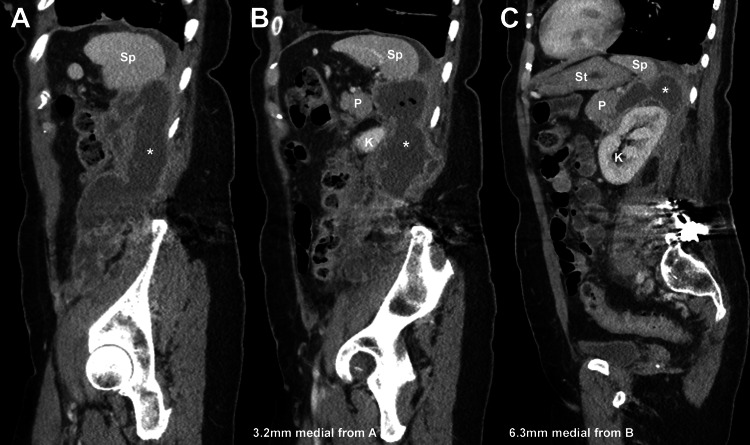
Sagittal CT images of our patient's abdomen. (A) The plane that includes the medial aspect of the left femoral head. (B) The medial aspect of the hip joint and is 3.2 mm medial from (A). (C) The sacrum is now seen because the image is 6.3 mm medial from (B). Sagittal CT images were obtained three months after our patient’s lumbar spine surgery (February 14, 2023). The pancreatic pseudocyst is indicated by the asterisk. P: pancreas; K: kidney; Sp: spleen; St: stomach.
